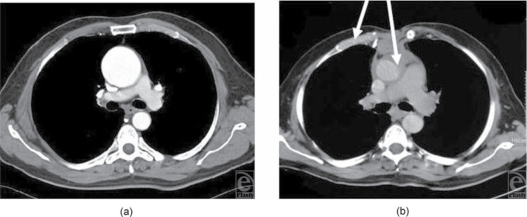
(a) Preoperative computed tomographic (CT) scan. (b) Postoperative (12 months) CT scan.
